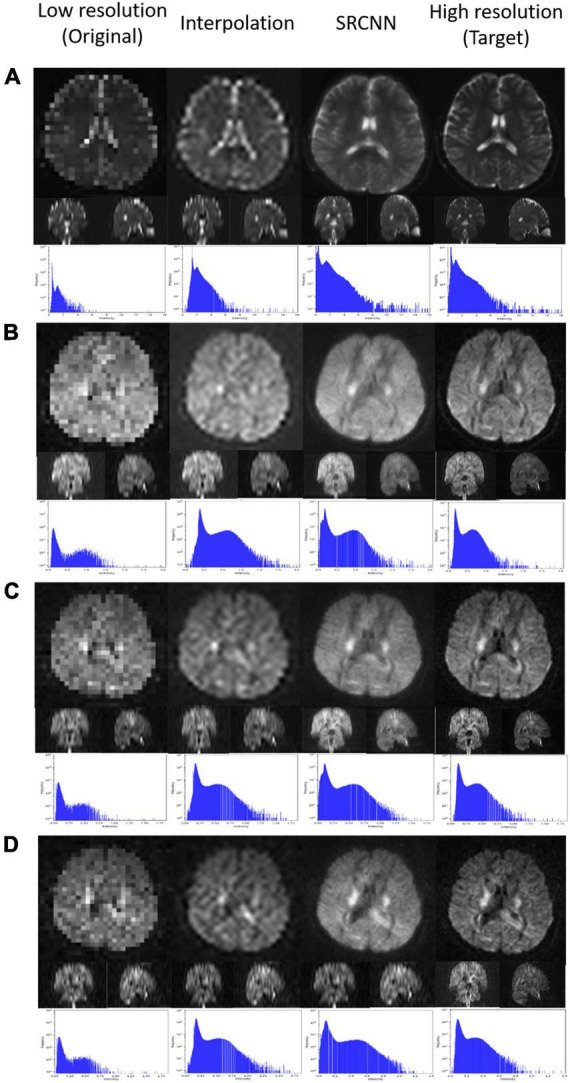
Super-resolution result of diffusion-weighted imaging (DWI) including (A) null; (B)
